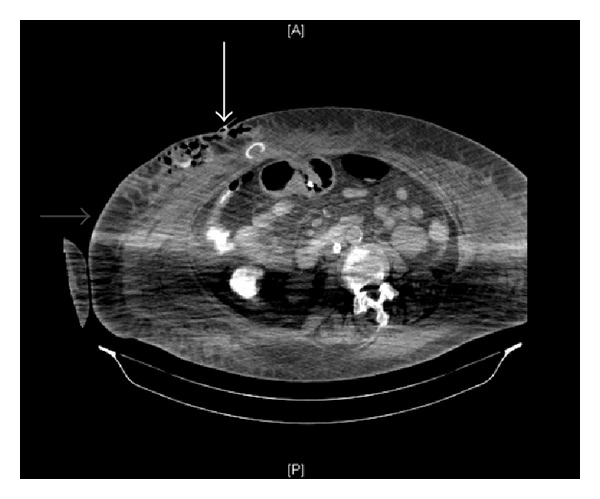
CT image of the abdomen. (1) The solid arrow indicates dislodgement of the internal bumper of the PEG tube into the abdominal wall outside the peritoneum. (2) The hollow arrow shows subcutaneous collection of fluid and air in the abdominal wall.
